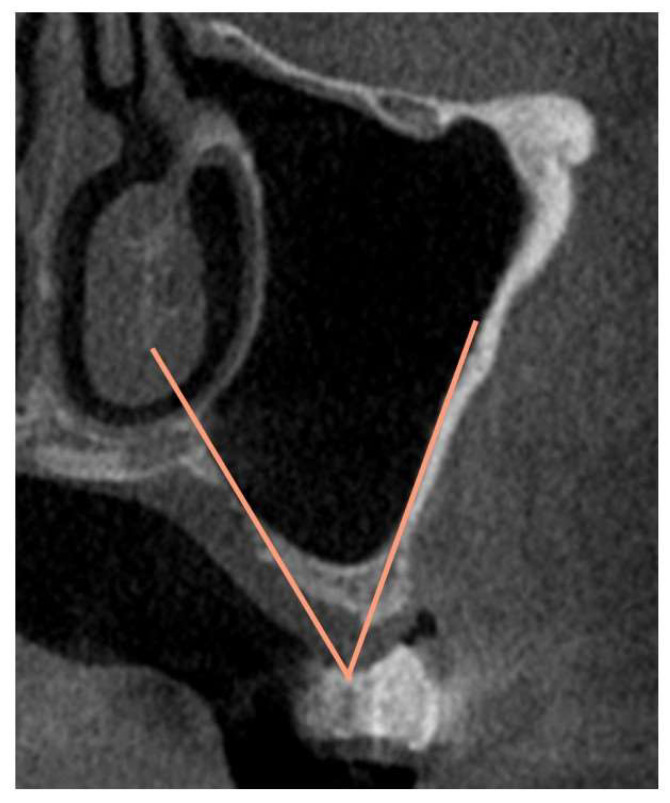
Maxillary sinus angle (between the lateral and medial wall of the maxillary sinus (orange line). Measurements were obtained within the plane perpendicular to the maxillary buccal bone of the tooth defect in the CT image.
